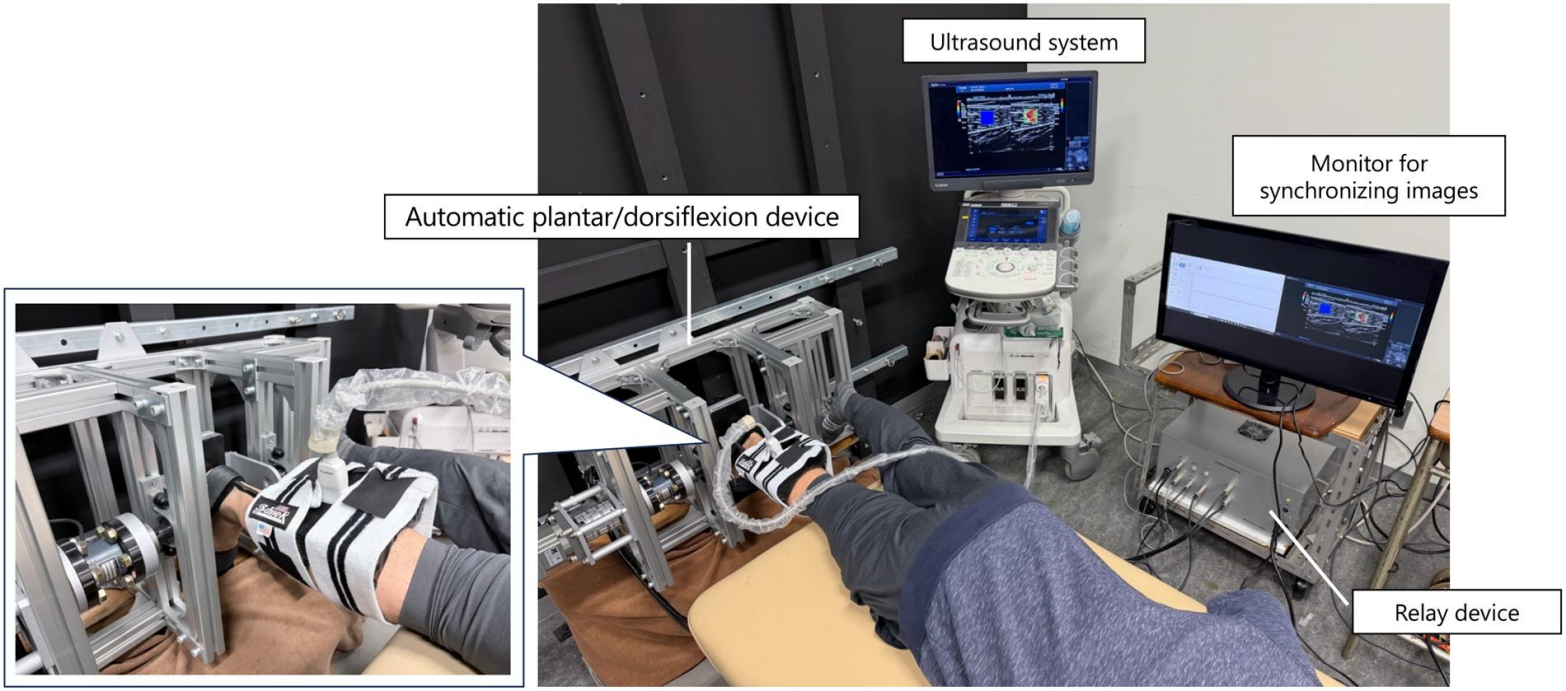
Experimental setup. Participant lying in the prone position on a dynamometer attachment, with the dominant foot secured by a belt. Ultrasound equipment was used to perform shear wave elastography (SWE) of the triceps surae and Achilles tendon (AT). For MG, LG, and SOL measurements, the ultrasound probe was fixed to the lower leg using a soft pad and elastic band to minimize movement and avoid probe pressure. For AT measurements, the probe was held manually due to interference with the dynamometer attachment. The dynamometer and ultrasound system were connected to a single display to synchronize shear wave velocity (SWV) with joint angle.
